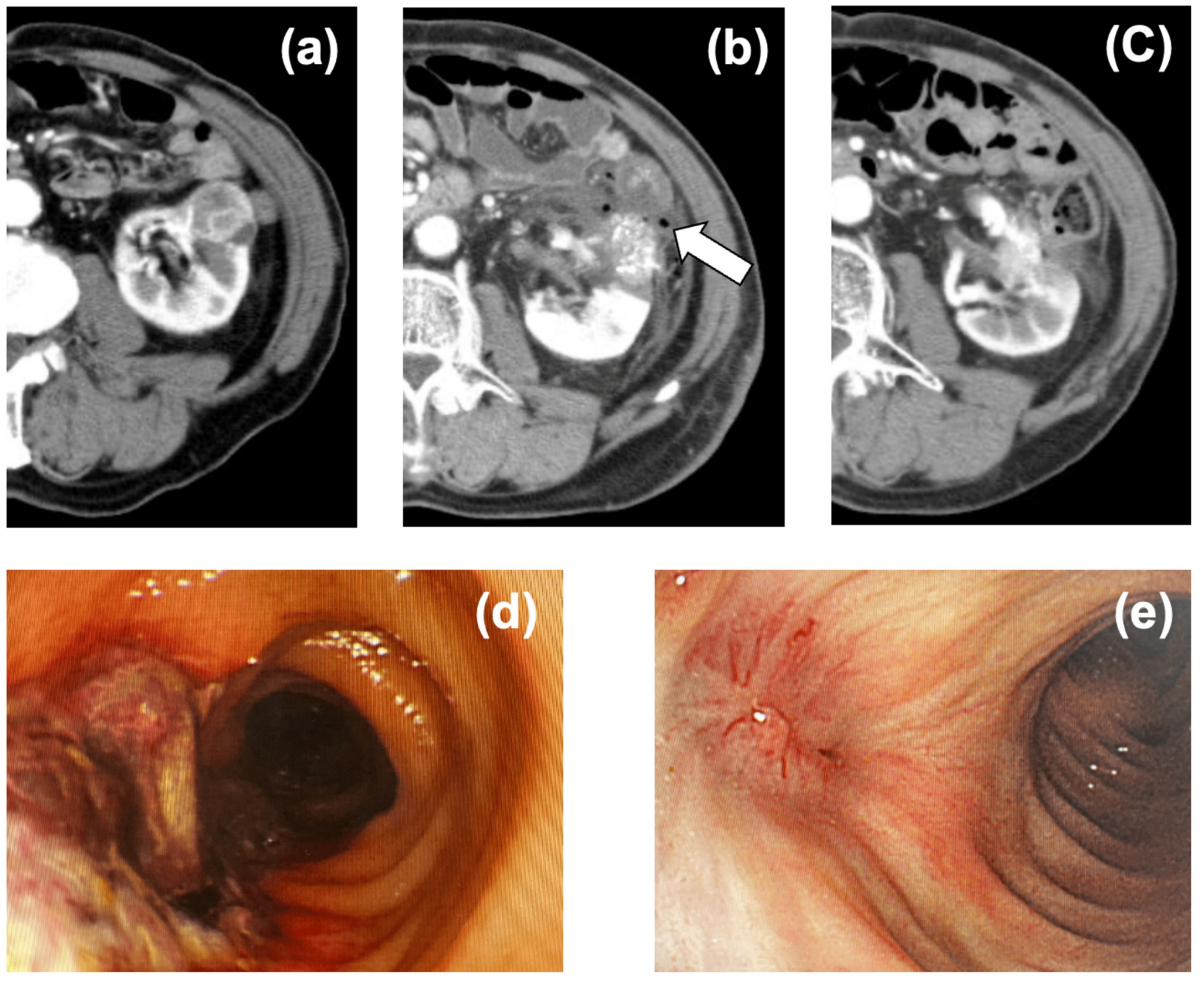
Case 1: Colorenal fistula following PCA (a) Contrast-enhanced CT showing a 38 mm exophytic renal cell carcinoma in the left kidney. (b) Post-procedural CT demonstrating an ischemic area of the colonic wall (arrow). (c) Follow-up CT four weeks later showing an improvement in the enhancement of the colonic wall. (d-e) Colonoscopy images demonstrating bowel wall ulceration at onset (d) and resolution after treatment (e). PCA: percutaneous cryoablation
